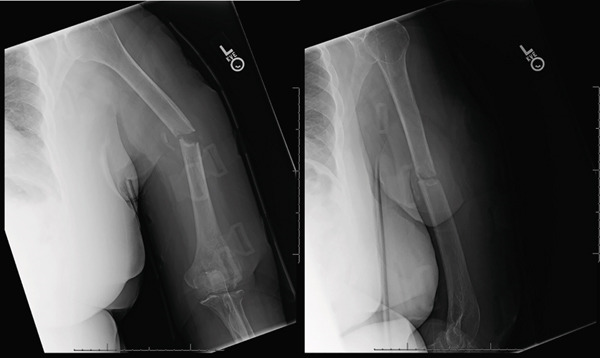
AP and lateral radiographs of the left humerus obtained 4 weeks after injury. There is approximately 35° of varus malalignment with some interval callus formation.
